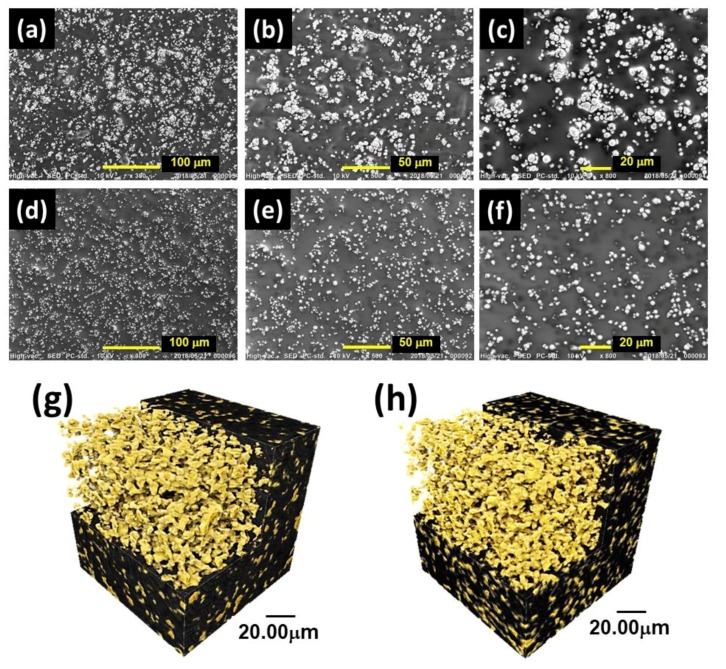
(a–f) SEM photographs and (g,h) computed tomography images for magnetic elastomers (a–c,g) without and (d–f,h) with sonication for 20 min.
